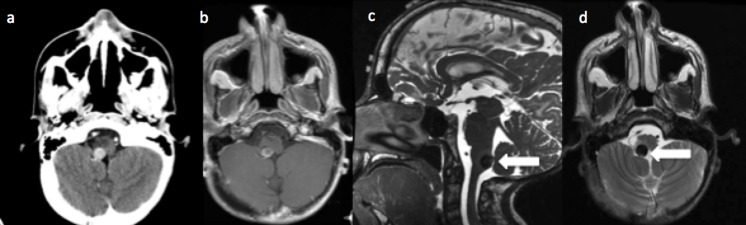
Presenting magnetic resonance imaging (MRI) after worsening hiccups (a) Computed tomography (CT) with contrast showing 1 cm enhancing lesion in R posterior medulla (40% increase in size from six weeks prior). (b) T1 with contrast axial MRI showing enhancing portion of cavernoma. (c) T2 high-resolution sagittal MRI depicting cavernoma (white arrow) location in lower posterior, right medulla. (d) T2 axial MRI showing the cavernoma (white arrow) with surrounding brainstem edema.
